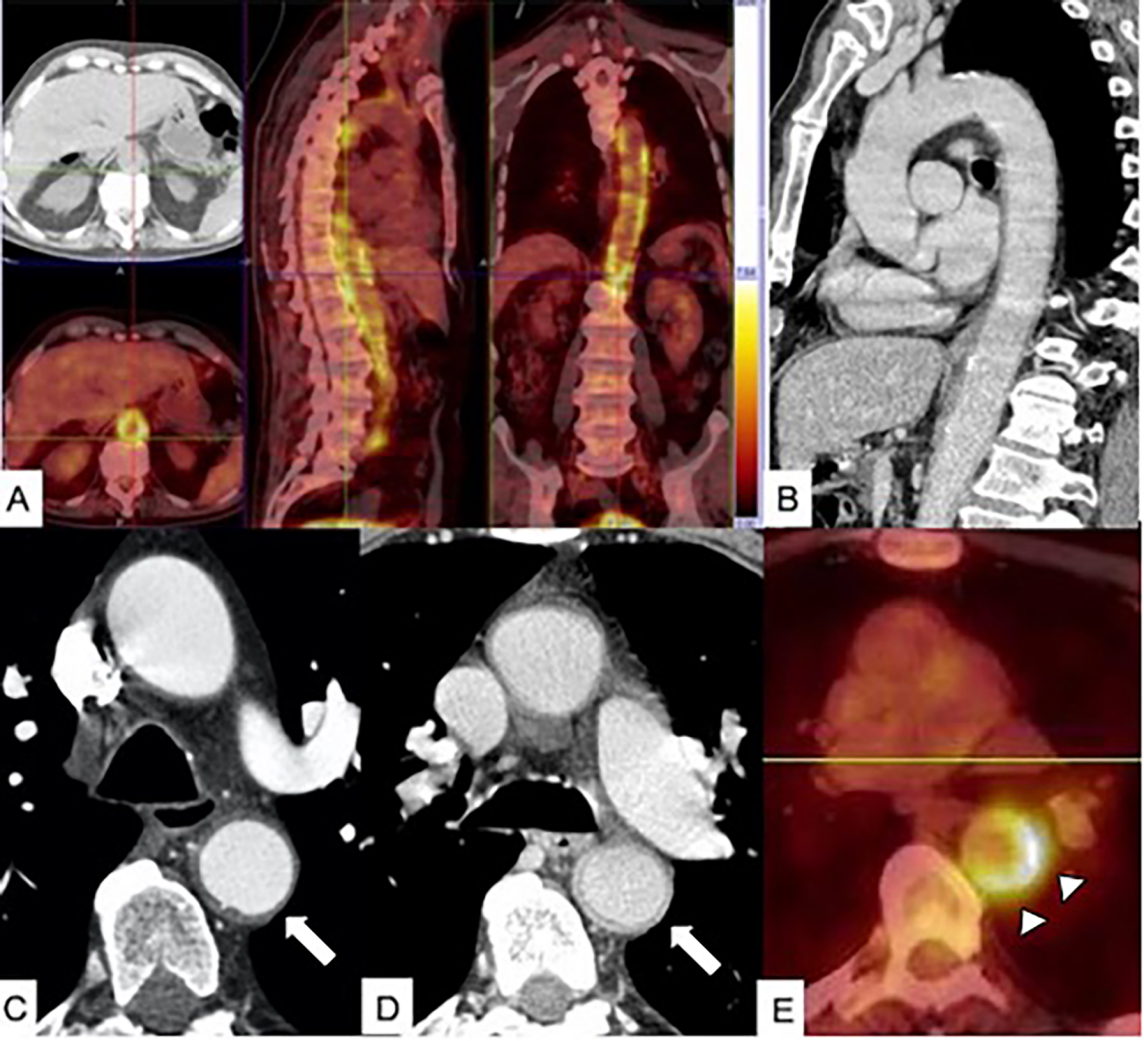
PET/CT in aortitis. (A) Axial PET/CT fusion image in a 68-year-old male patient with diffuse aortitis showing an [18F]-fluorodeoxyglucose (FDG) uptake in the thoracoabdominal aortic wall (arrow). (B) Sagittal multiplanar reconstruction CT image acquired in the portal phase shows thickened aortic wall enhancement. In a different 70-year-old female patient with giant cell arteritis, arterial-phase CT scan (C) shows thickening of the descending aortic wall mimicking an IMH (arrow), and venous phase CT image (D) shows enhancement of thickened aortic wall (arrow). (E) Axial PET/CT fusion image in the same patient shows FDG uptake of the descending aorta wall (arrowheads).
